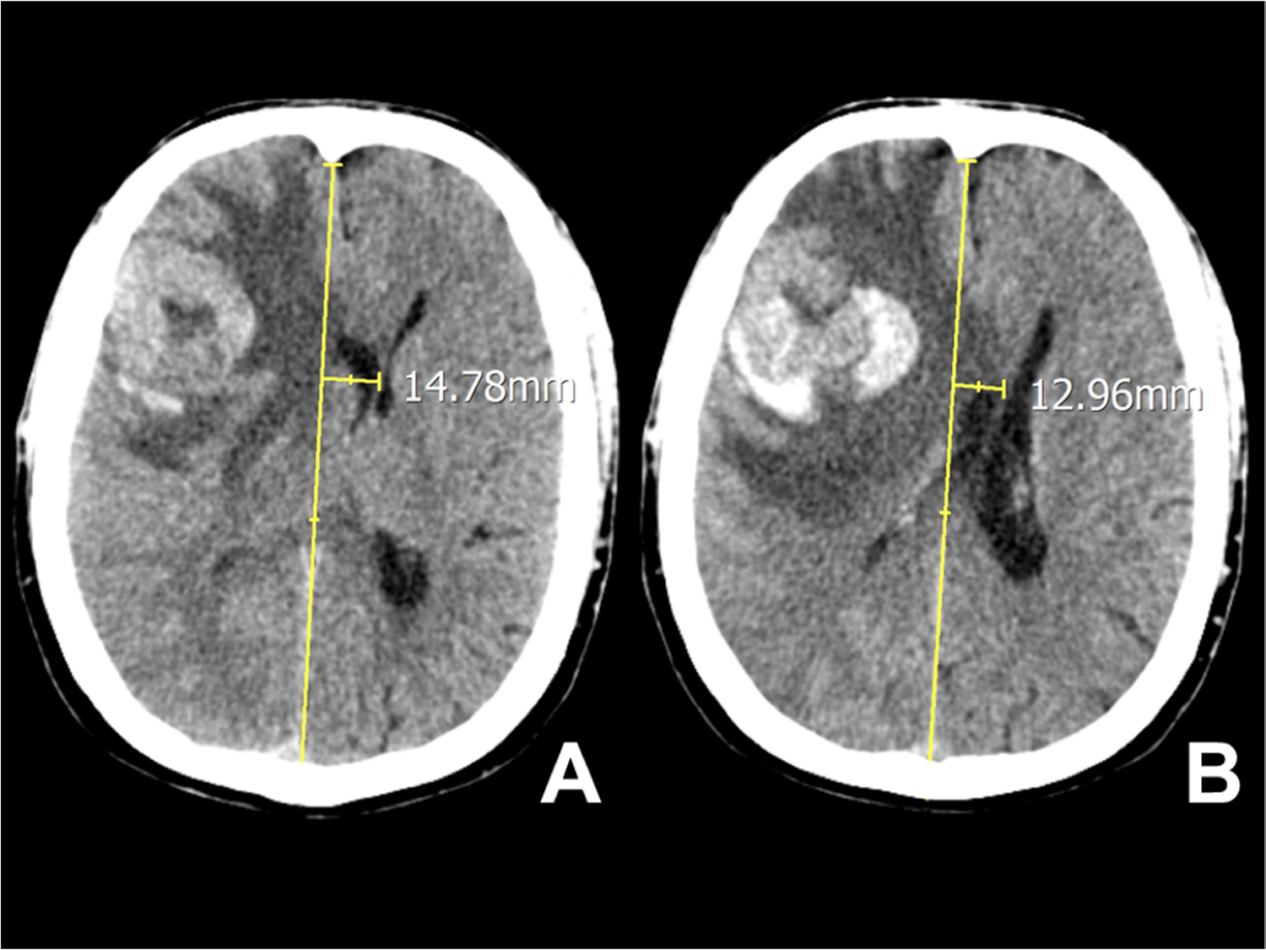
In a patient with a hemorrhagic tumor, brain shift was evaluated in the axial CT scans at the level of MF (A). Both frontal horns are displaced from right to left. Using the CT software, a sagittal line (drawn from the crista galli and anterior cerebral falx to the internal occipital protuberance) is intersected by a perpendicular transverse line starting from the septum pellucidum. The midline shift was measured on the transverse line, in millimeters. The same procedure is performed at the CC (B)
